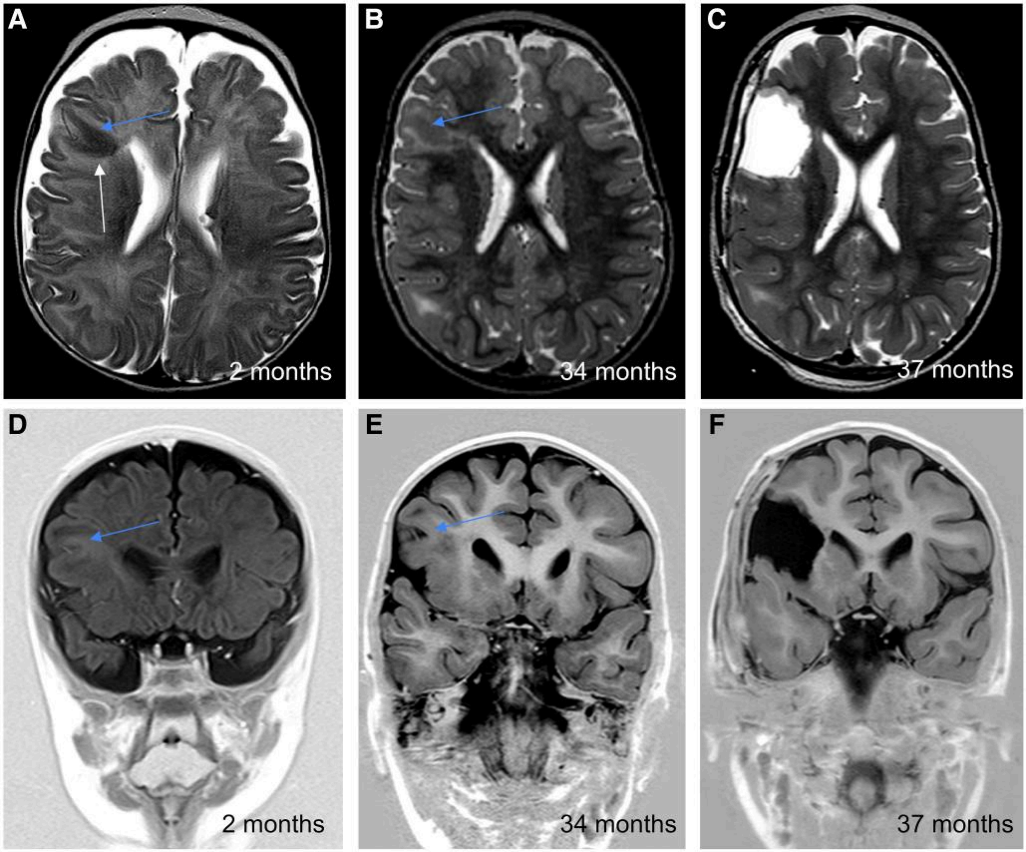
Example of evolution of a T2HI lesion on unmyelinated brain MRI to a highly dysplastic lesion on myelinated brain MRI. These MRI images show the evolution of T2-hypointensity (white arrow) in the right fronto-opercular region. Axial T2-weighted images at 2 months (A, unmyelinated) and 34 months (B, myelinated), with corresponding coronal T1-weighted images (D and E). Note the persistent cortical thickening (blue arrows). Post-surgical images (C, axial T2; F, coronal T1) at 37 months confirm complete lesion resection. The patient has remained seizure-free since surgery. The patient is seizure-free since surgery.
